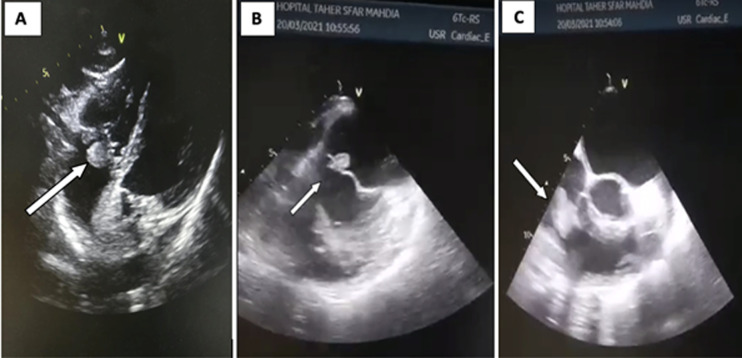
A) huge vegetation at the expense of the tricuspid valve in the subcostal window (15*20mm); B) vegetation at the expense of the mitral valve in transesophageal echocardiography (7*4mm); C) vegetation at the expense of the Tricuspid valve (15*20mm) in the transesophageal echocardiography (TEE)
